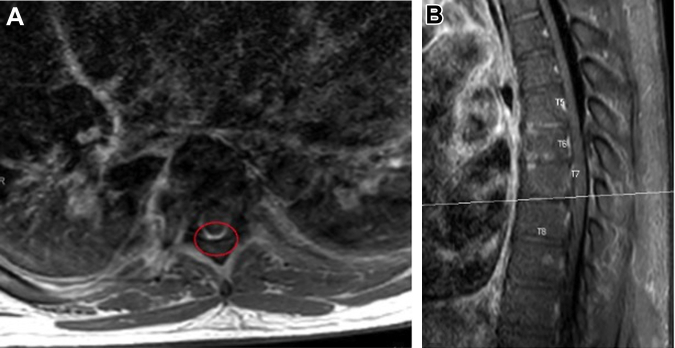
MRI of Thoracic Spine With Epidural Abscess (A) Axial postcontrast T1-weighted MRI at T7 and T8 with posterior epidural enhancement consistent with early epidural abscess formation (red ellipse). (B) Correlating sagittal postcontrast T1-weighted MRI with evidence of spinal epidural abscess formation at T7 and T8. MRI = magnetic resonance imaging.
